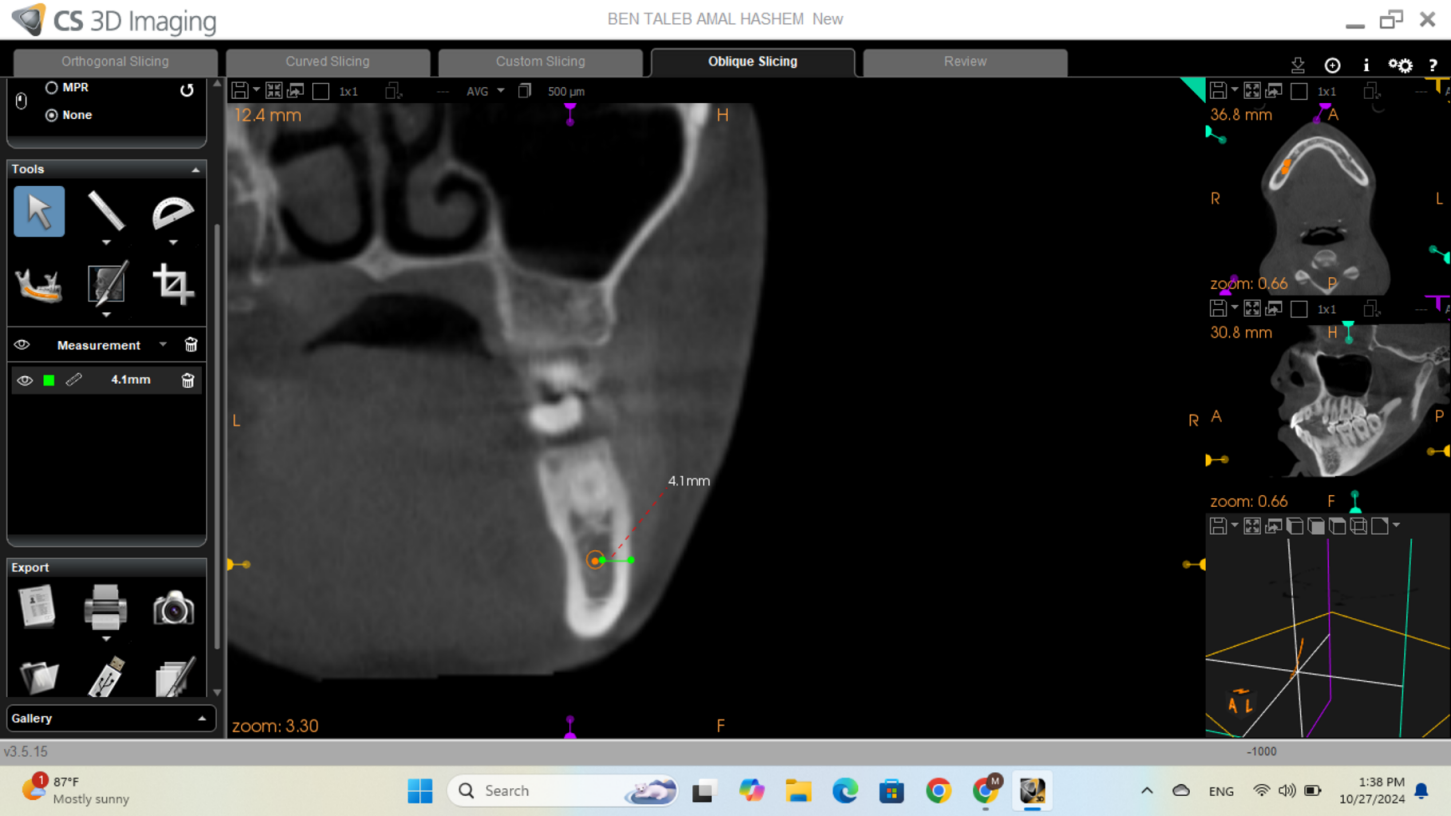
Cross-sectional view of CBCT for buccal cortex thickness measurement.
